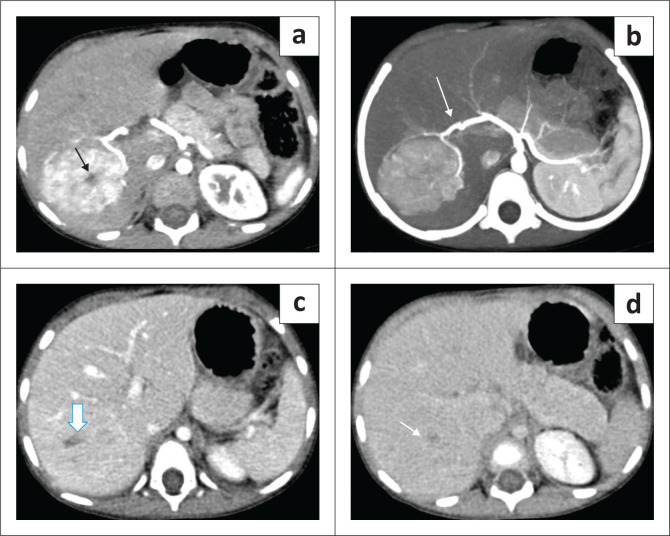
Focal nodular hyperplasia in a 5-year-old child. Dynamic contrast-enhanced CT scan of the abdomen showing an arterially enhancing mass in the right lobe of the liver with a hypodense central scar (black arrow in a). Maximum intensity projection image shows arterial feeders from the right hepatic artery (arrow in b). Persistent enhancement is seen in the lesion on the portal venous (c) and the delayed phase (d) shows some enhancement of the central scar.
